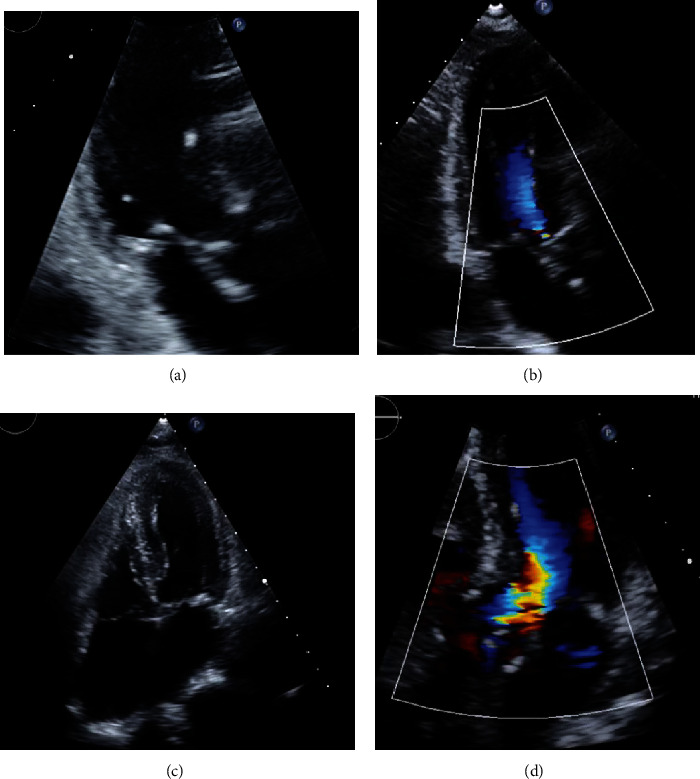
The echocardiogram performed on 4^th^ day postoperatively showed complete relief of left ventricular outflow tract (LVOT) obstruction. (a) No SAM was seen on the three-chamber view. (b) No flow acceleration nor mitral regurgitation on the three-chamber view with color mapping. (c) Patent LVOT and regular LV shape. (d) No flow acceleration nor mitral regurgitation on the five-chamber view with color mapping. The Supplement video 3 and Supplement video 4 provide a better appreciation of normal LV shape, good LV contraction, and patent LVOT.
